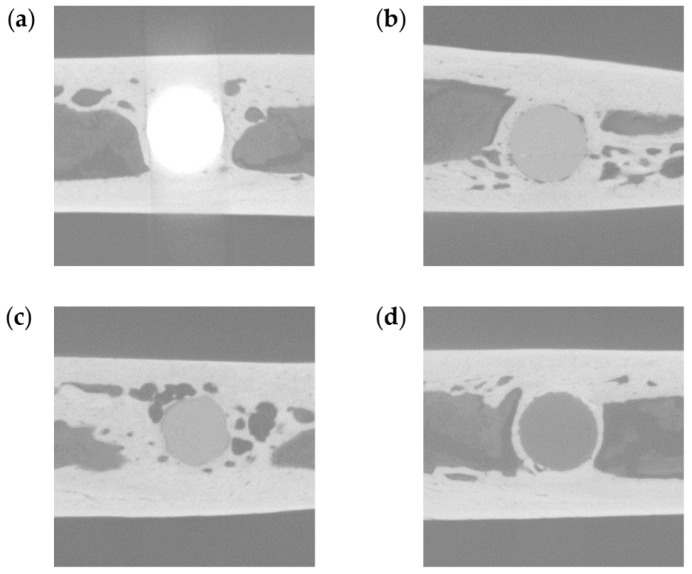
Representative micro-computed tomography (CT) images. (a) TiA, (b) MgA, (c) Mg, and (d) PLA implants.
